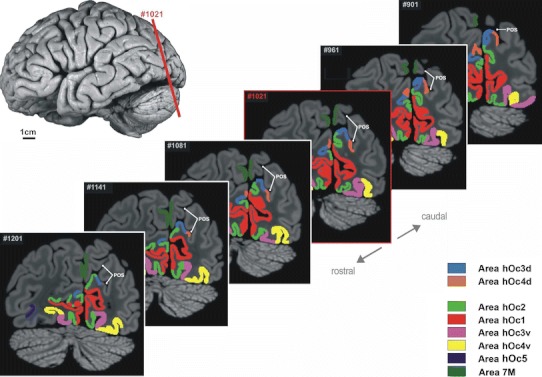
Mapping of areas hOc3d and hOc4d as well as their neighboring areas (Amunts et al. 2000; Malikovic et al. 2007; Rottschy et al. 2007; Scheperjans et al. 2008) in six MRI coronal sections of an individual post-mortem brain. The *red line* in the upper left image shows the position of section 1021. Identified areas are labeled by different colors. *POS* parieto-occipital sulcus
